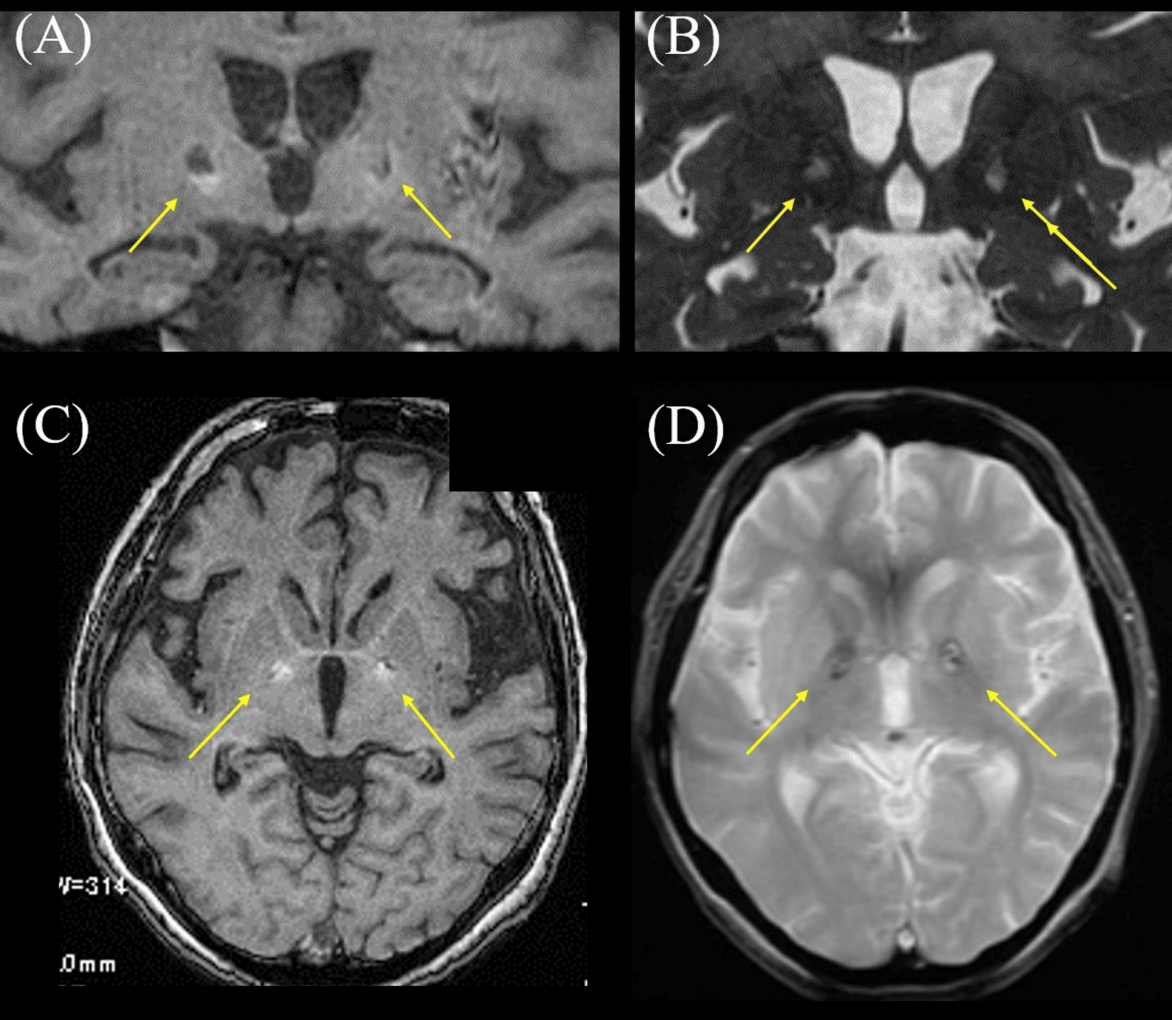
The result of brain MRI 10 months after onset. (A,C) T1-weighted brain MRI showing BPL with mixed hyperintensities and hypointensities on T1-weighted imaging. (B) T2-weighted brain MRI showing BPL with hyperintensities. (D) T2*-weighted brain MRI showing BPL with patchy hypointensities. MRI, magnetic resonance imaging; BPL, bilateral globus pallidus lesions
